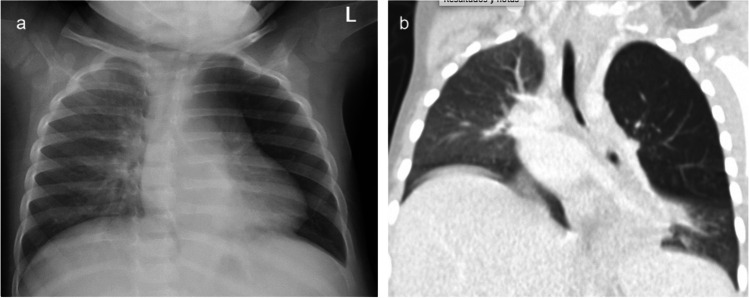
Radiographs of an 18-month-old girl. a The anteroposterior (AP) view indicates the left lung appears more radiolucent than the right, with a slight diminution in lung markings. There is a suspicion of a foreign body in the left main bronchus causing a so-called interrupted bronchus sign. b Coronal view computed tomography (CT) with contrast, in lung window, confirms the presence of an inhaled foreign object in the left main bronchus, which has resulted in air trapping in the left upper lobe
